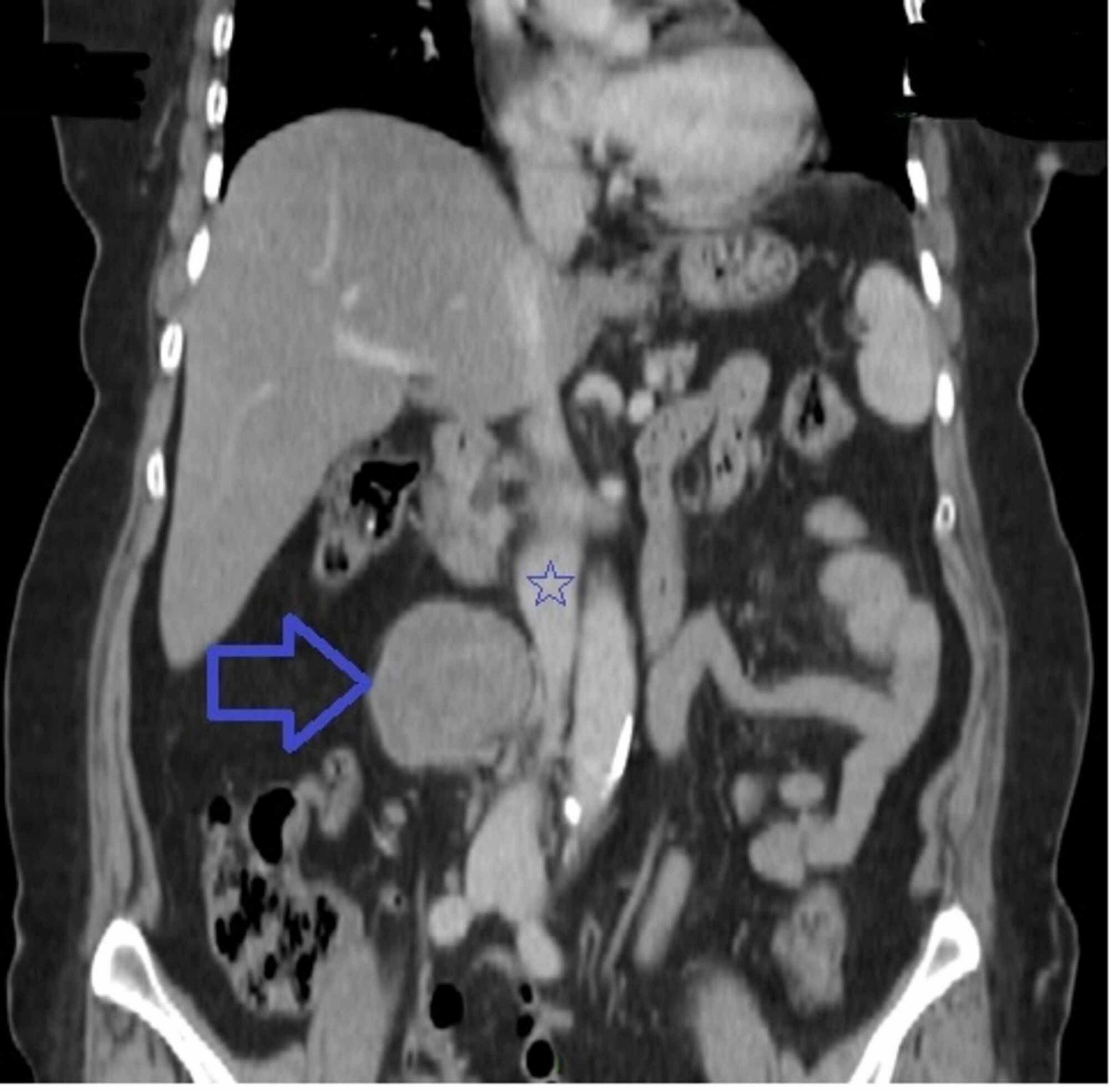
Coronal abdominal CT scan showing a retroperitoneal mass (blue arrow) located in the lateral and retrocaval area with intimate contact with the inferior vena cava (blue star).
